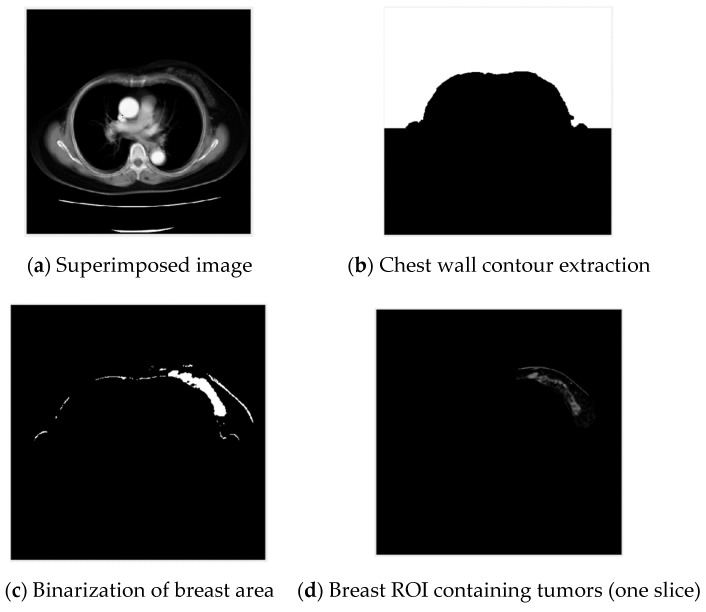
Chest wall contour extraction of CT images.
